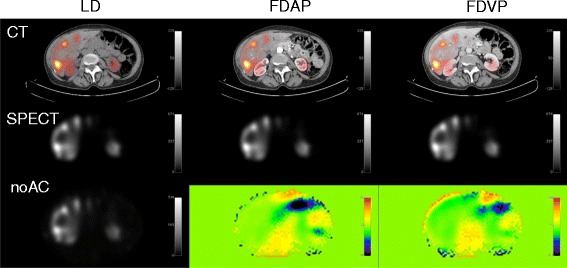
CT images. Top row: CT images for LD, FDAP, and FDVP. Middle row: AC-SPECT images for LD, FDAP, and FDVP. Lower row: first image noAC-SPECT images; second images percent difference between LD- and FDAP-SPECT; third image percent difference between LD-FDVP-SPECT.
